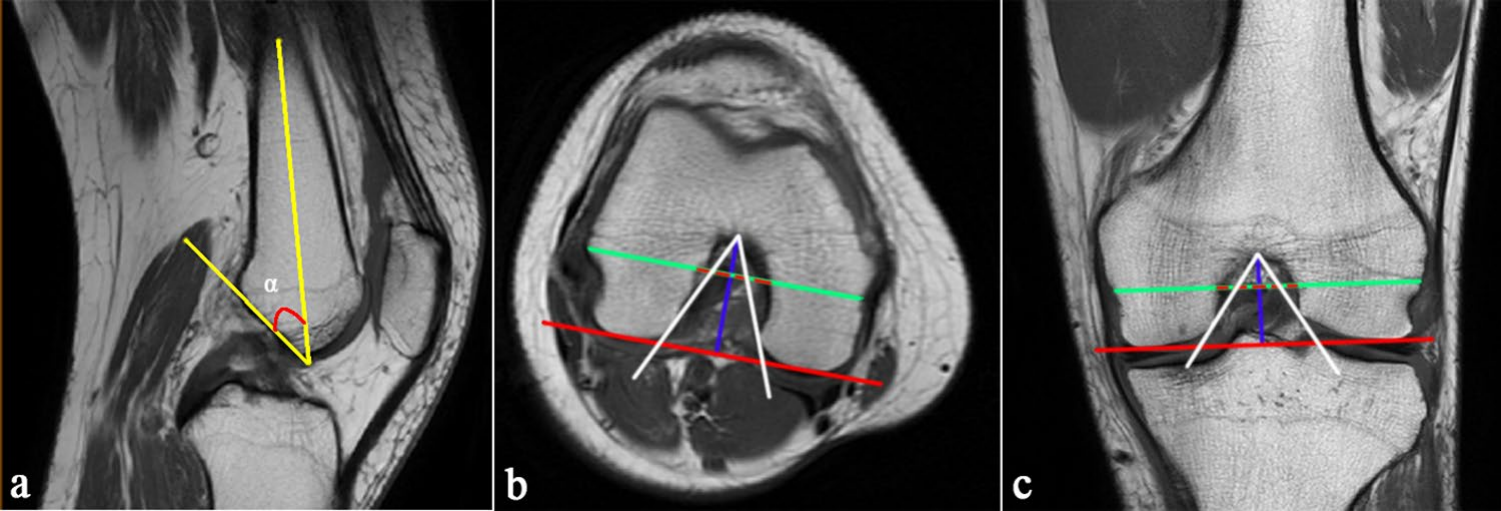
Morphological measurements of the femoral condyle. a Sagittal MRI image of the knee, showing the ‘*α*’ angle measurement. b Axial MRI image of the knee. The solid red line represents the distal end of the femoral condyles. The blue line represents the intercondylar depth. The green line represents the femoral condylar width. The dashed red line represents the intercondylar notch width. The intercondylar is measured between the two white lines. c Coronal MRI image of the knee. The measurement methods are same as b
